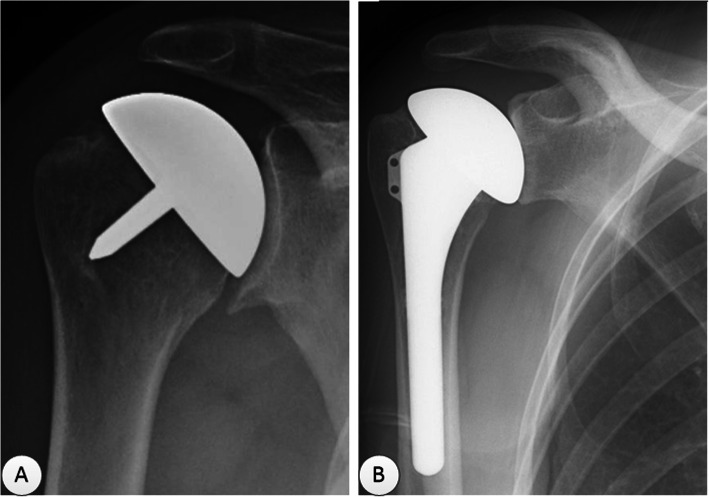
Anteroposterior radiographs (postoperation) for the treatment of GHOA. a resurfacing hemiarthroplasty (RHA); b stemmed hemiarthroplasty (SHA)
